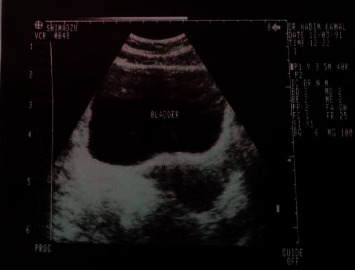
Ultrasound scan of the kidneys, ureters, and bladder showed thickening and irregularity of the bladder wall.
